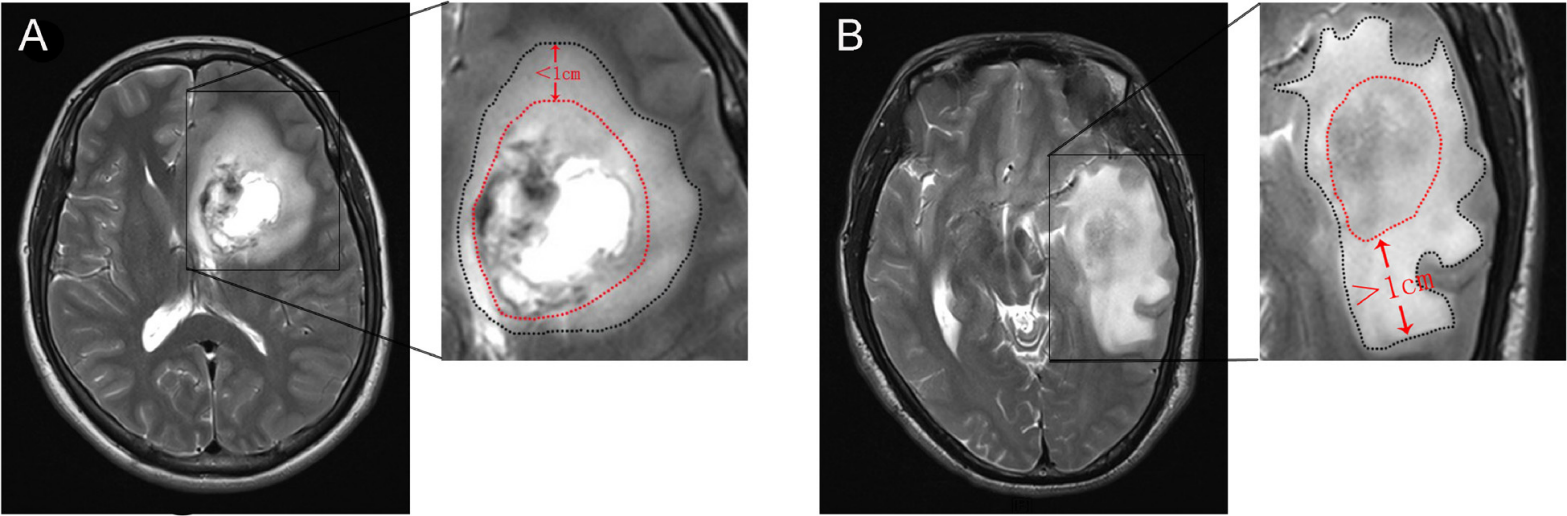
Eveluation of PTE. A region of very bright T2-W signal surrounding the tumor, which was estimated on the base of the maximum distance from the tumor margin to the outer edge of edema. (A) Minor edema (<1 cm) shown by T2-W MRI. (B) Major edema (>1 cm) shown by T2-W MRI.
